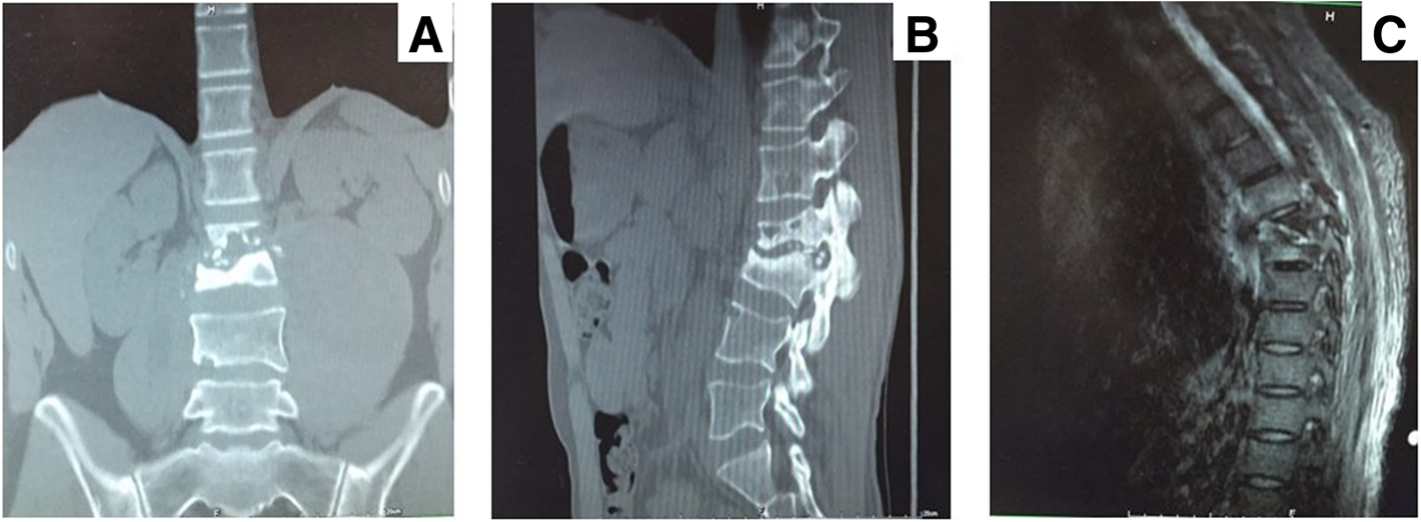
CT and MR of spine tuberculosis. a CT of spine TB: anterioposterior position; b CT of spine TB: lateral position; c MR of spine TB
